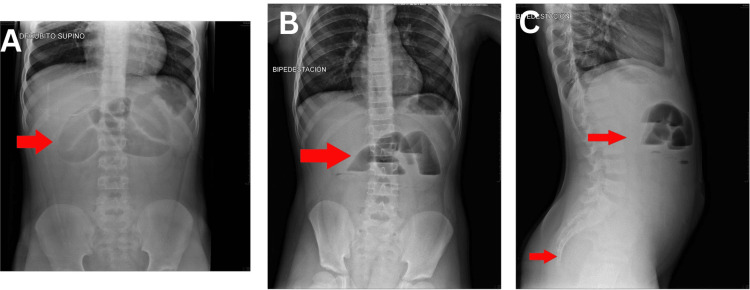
Abdominal X-ray A: supine view showing diffuse small-bowel distension (arrow); B: upright view demonstrating multiple air-fluid levels with paucity of distal gas (arrow); C: lateral upright view confirming air-fluid levels and absent rectal gas (arrows).
